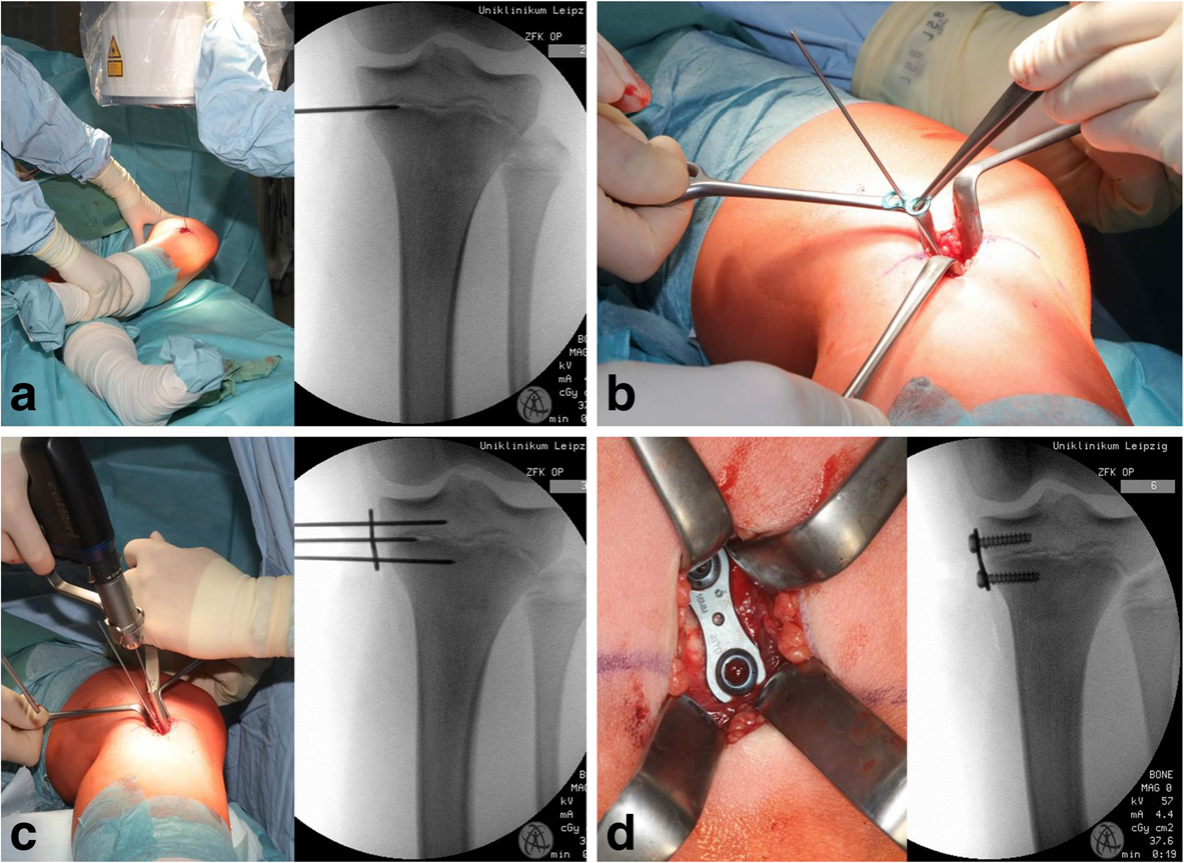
Surgical field during the implantation of a proximal medial tibial eight-Plate: a: marking of the growth plate using a K-wire with the aid of X-ray imaging; b: the eight-Plate implant is inserted through the corresponding hole and placed in the middle of the plate via the K-wire lying in the growth plate; c: the cancellous screws are then positioned using previously positioned K-wires; d: the inserted eight-Plate after implantation
